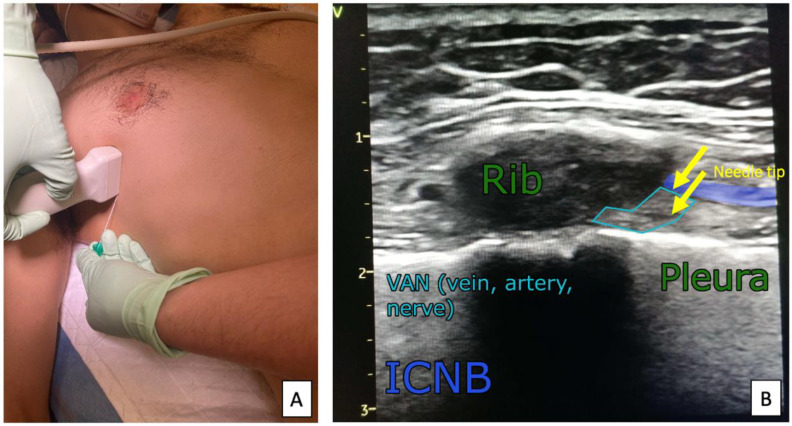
Ultrasound transducer positioning (A) and sonoanatomy of the ICNB block (B).
